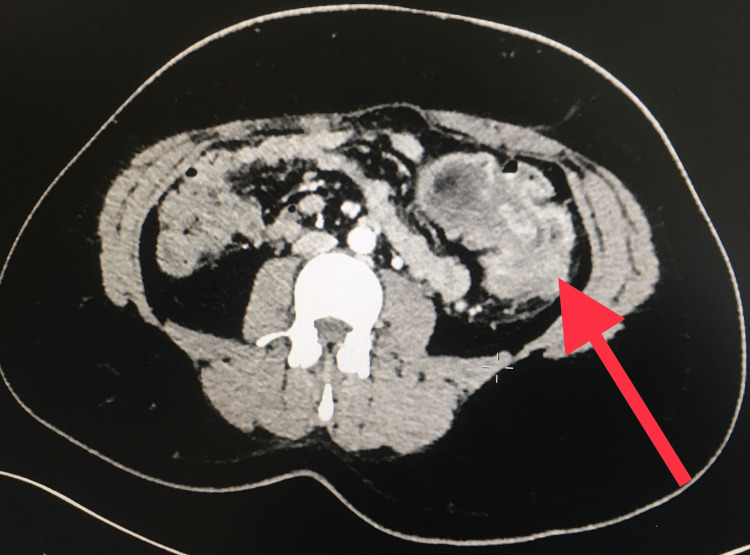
Axial view of the CT scan of the abdomen and pelvis Red arrow: Fat density on the intussusception of the descending-sigmoid colon
